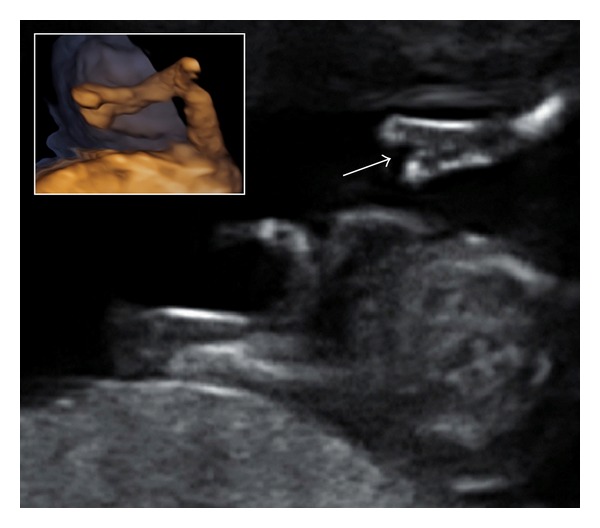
Ultrasound examination at 11 + 5 weeks' gestation: cleft hand (arrow) and three-dimensional view of the upper limb with associated radial agenesis.
